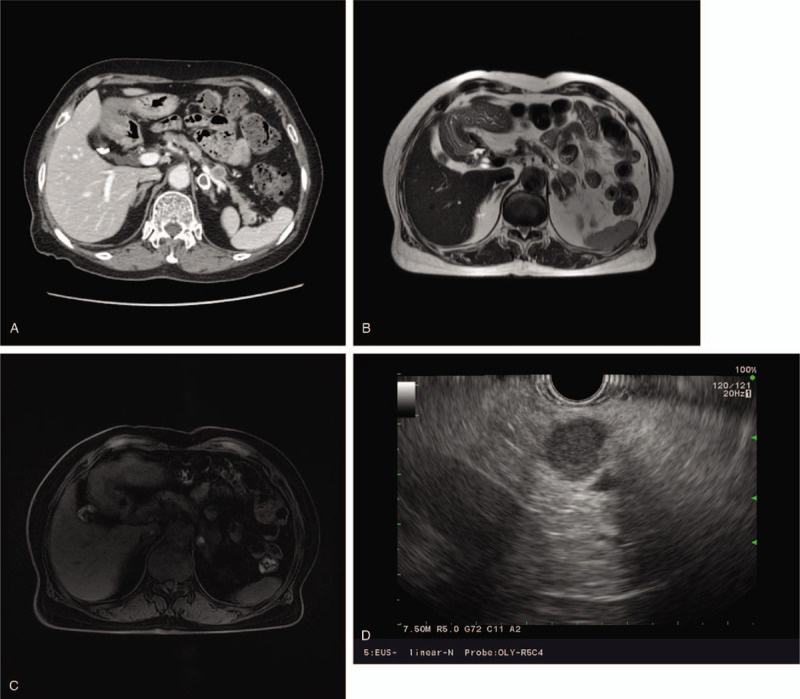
(A) Dynamic computed tomography image of the pancreas shows a mass in the tail of the pancreas mass. Dynamic contrast-enhanced magnetic resonance imaging of the pancreas shows a mass with (B) subtle high signal intensity on T2WI whereas (C) iso signal intensity on T1WI. (D) Diagnostic endoscopic ultrasonography fine needle aspiration of mass was performed.
